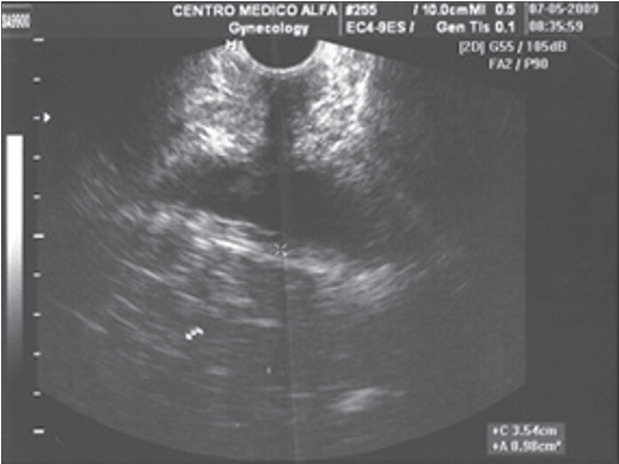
Ultrasound image of cross-sectional area of levator ani muscle.
